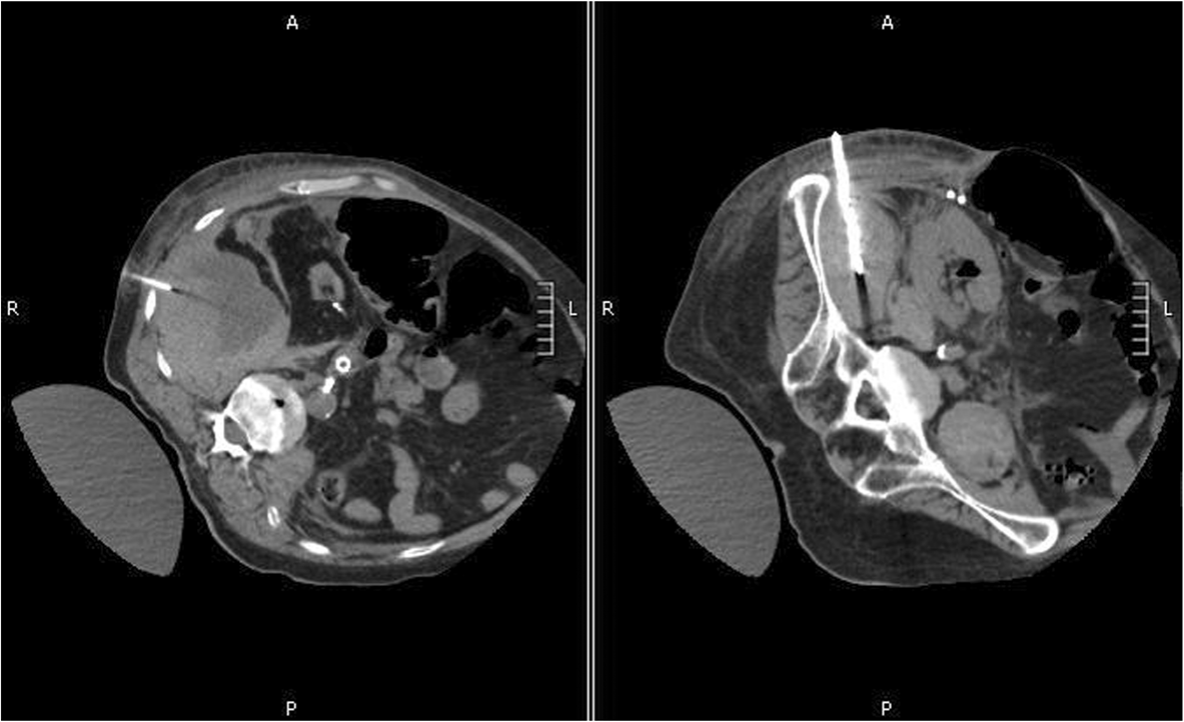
Computed tomography showing interventional radiology-guided drainage of the right retroperitoneal hematoma
